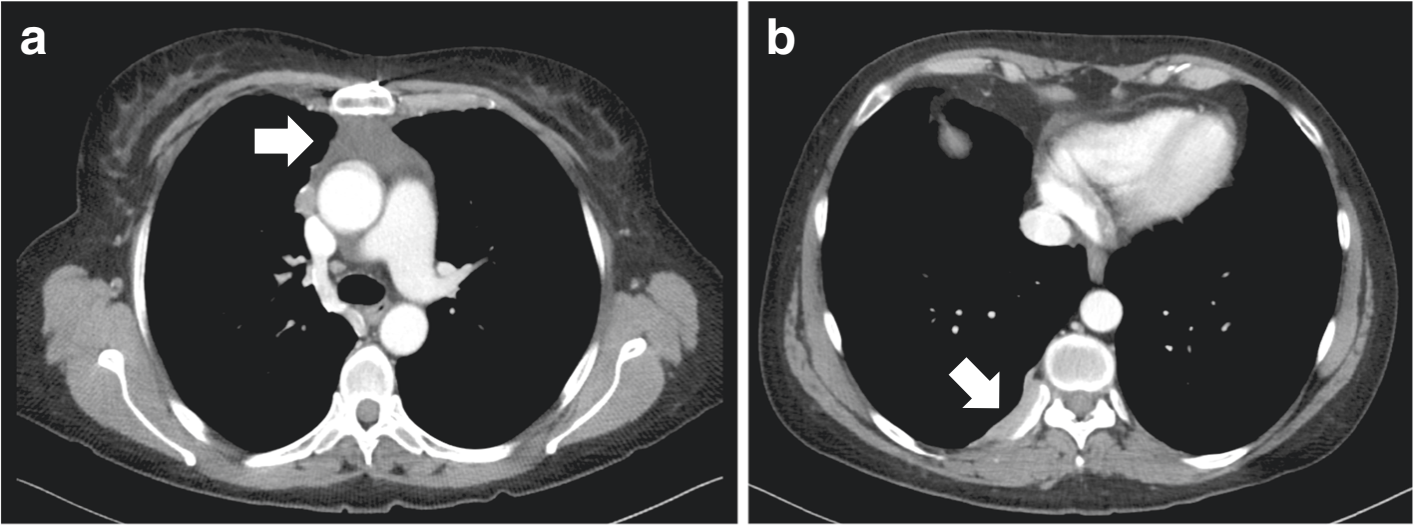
Metastatic thymoma on Computer Tomography (CT) of the chest. Evidence of metastatic thymoma at during follow-up as shown by (a) anterior mediastinal mass and (b) right sided pleural deposit
